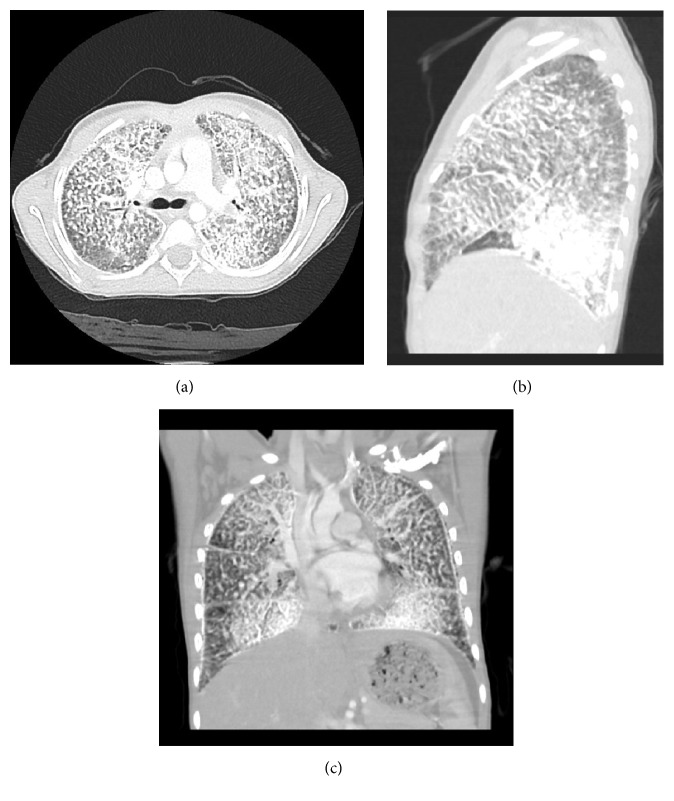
CT. (a) Axial CT image. (b) Sagittal CT image. (c) Coronal CT image.
